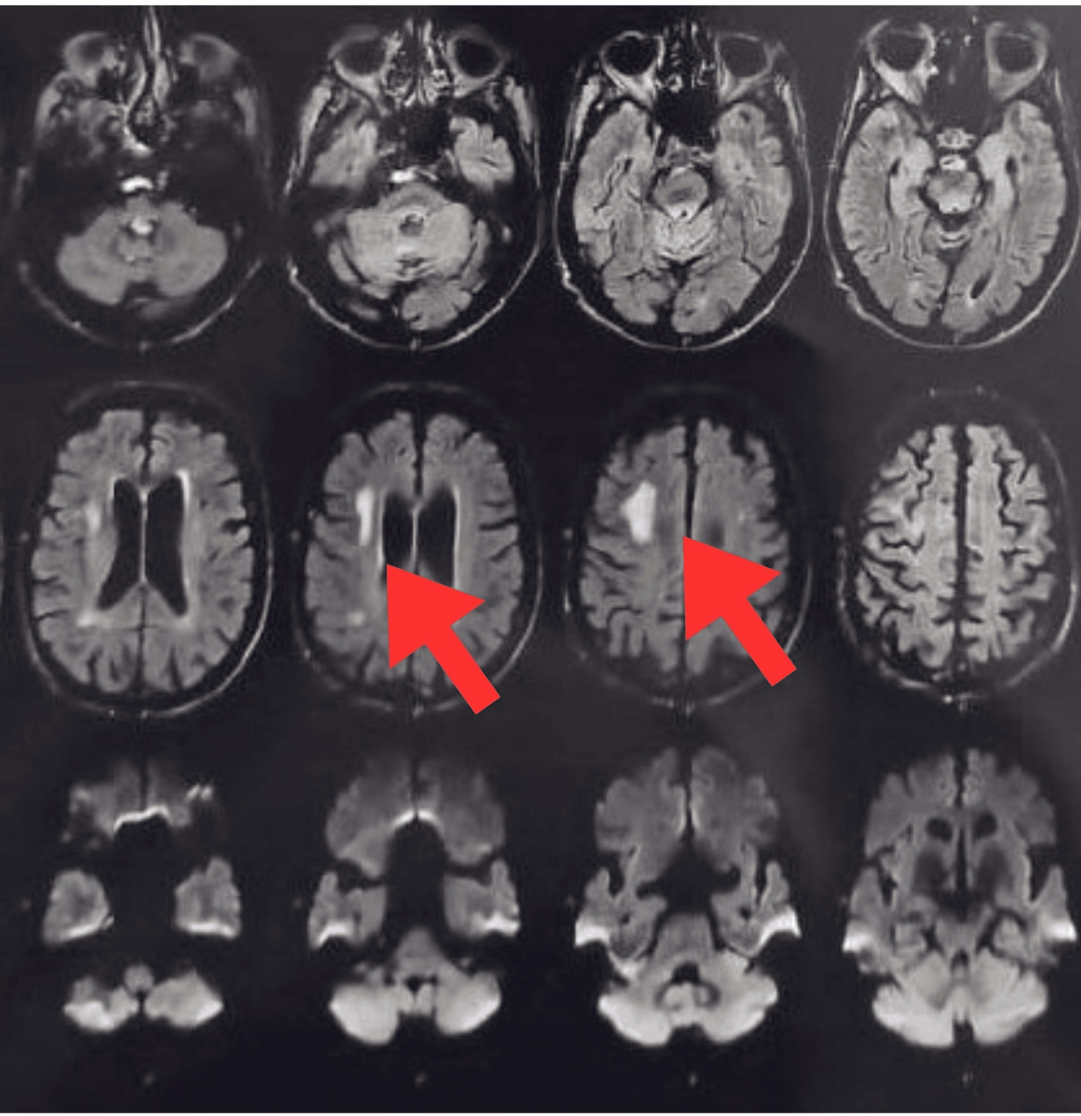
MRI shows ischemic changes
